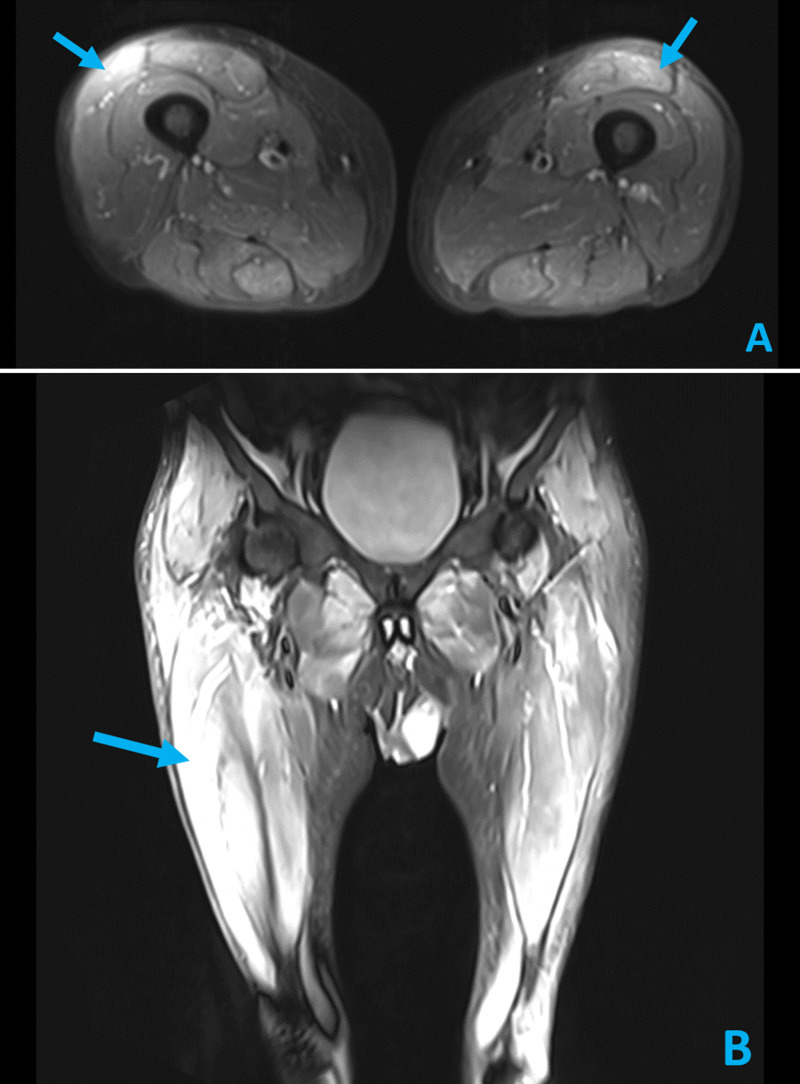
Axial (A) and Coronal (B) STIR MRI images of the lower extremities demonstrating bilateral, multi-compartmental myositis (arrows) with severe involvement of rectus femoris, semimembranosus, and adductor musculature. STIR, Short Tau Inversion Recovery
